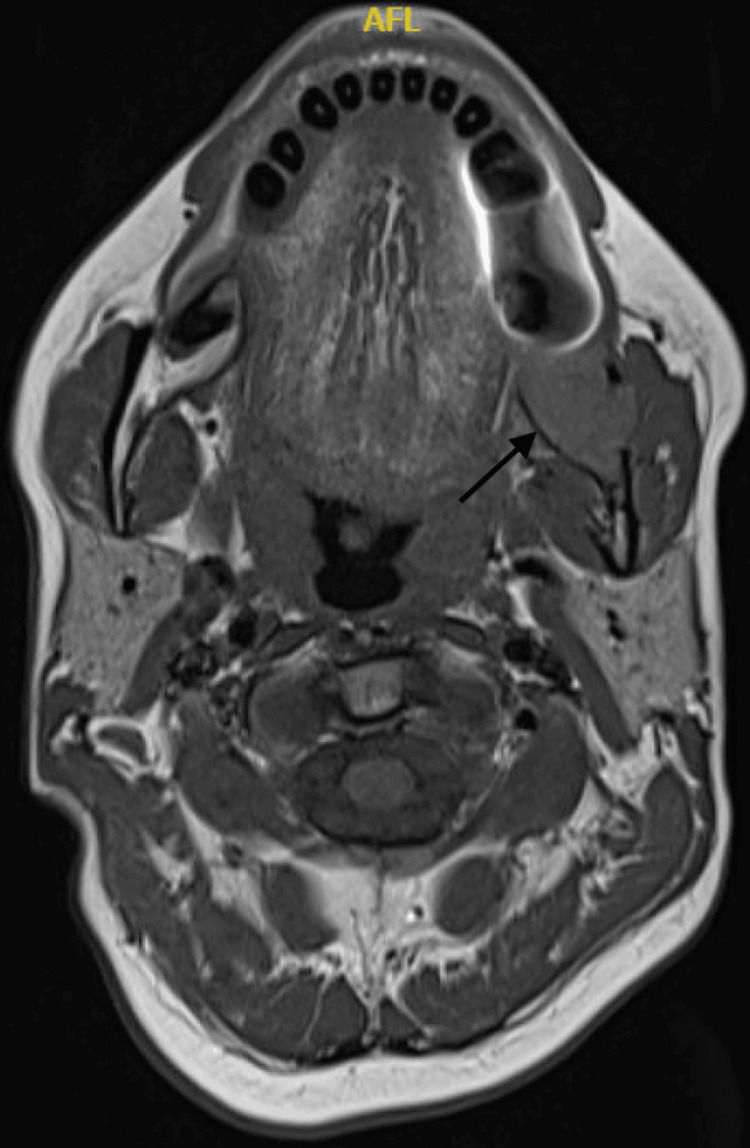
MRI of the left mandibular angle lesion with metastatic characteristics
